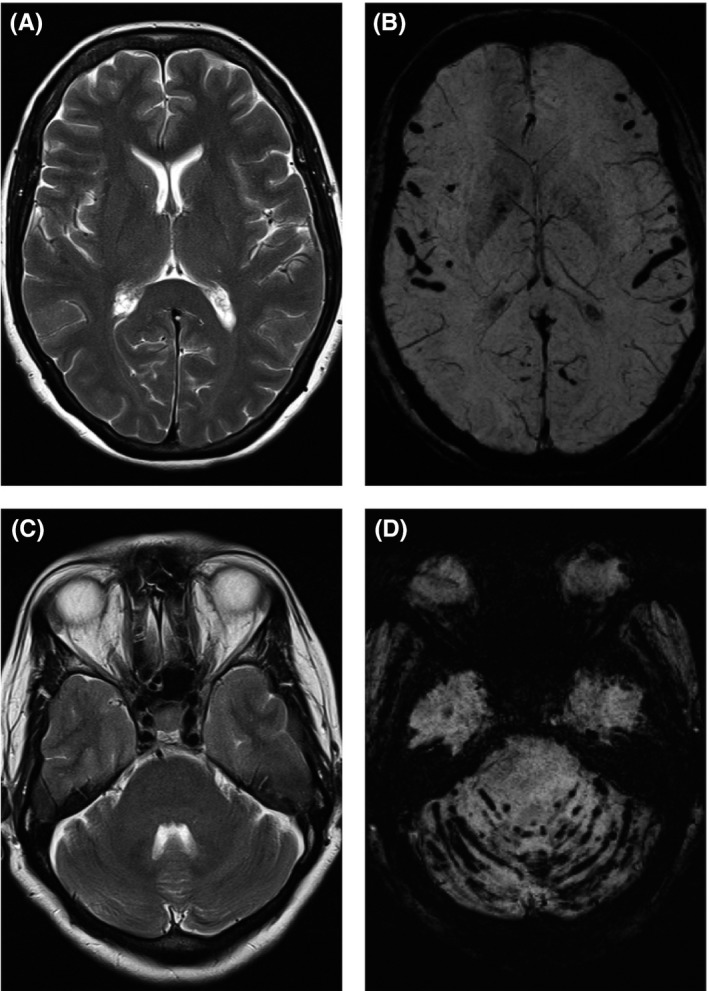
MRI head. Selected axial T2 weighted images of the supratentorial (A) and infratentorial (C) compartment that appear normal. However, the corresponding axial susceptibility weighted images (B and D) demonstrate extensive supratentorial and infratentorial blooming artifact reflecting sulcal pial hemosiderosis
